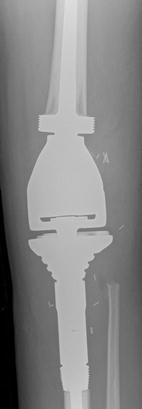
Post-resection AP plain radiograph demonstrates a Stanmore prosthesis with integral distal femur and modular proximal tibial component
